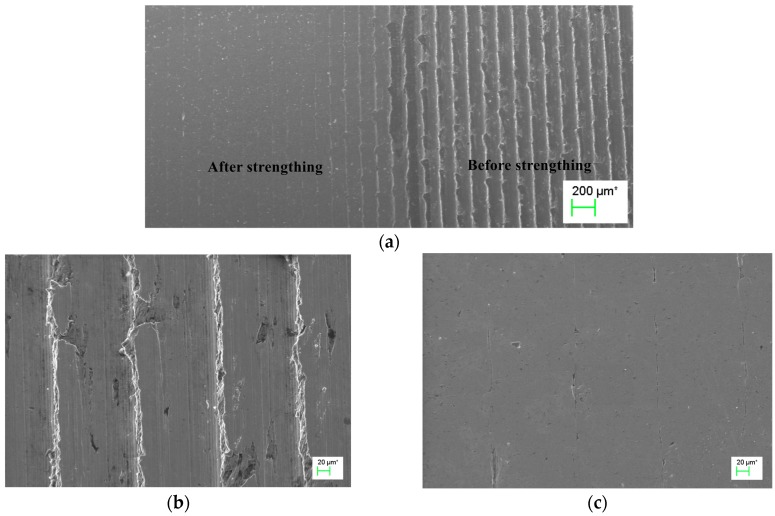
Appearance contrast before and after ultrasonic surface strengthening. (a) Appearance contrast before and after strengthening (20×); (b) Before strengthing (200×); (c) After strengthing (200×).
